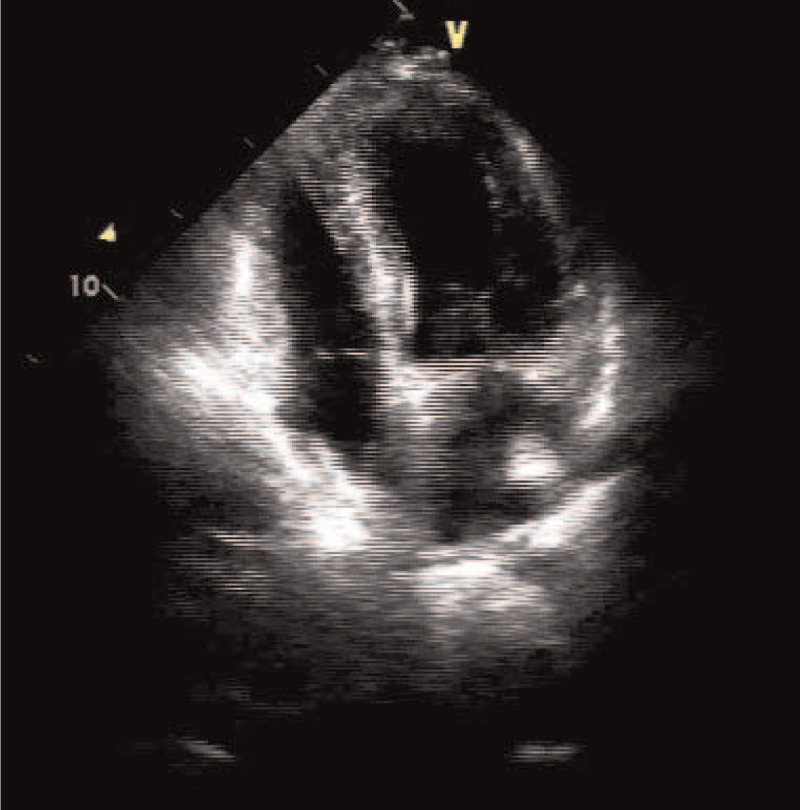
Transthoracic echocardiography demonstrates a 10 × 15 mm mobile hyperechoic mass arising from the opening of the left inferior pulmonary vein in left atrium.
